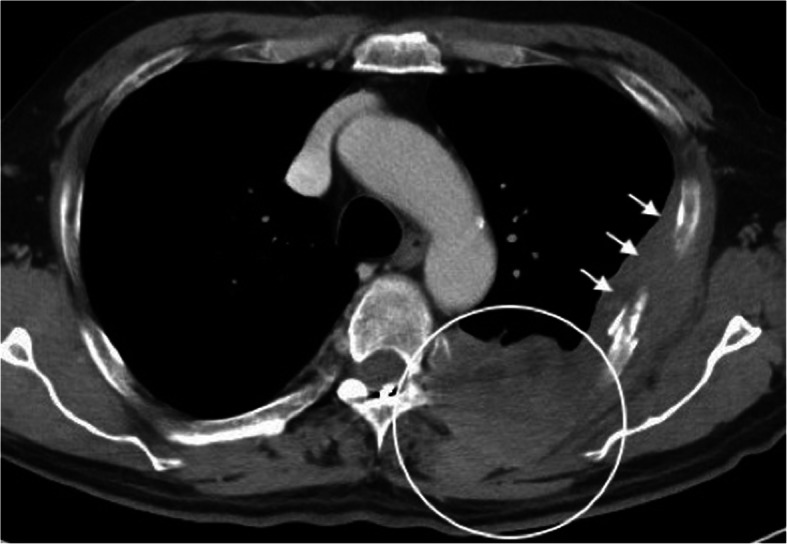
Axial slice of a CT scan showing a heterogeneous mass infiltrating the posterior thoracic tissue (in the white circle), and pleural thickening (white arrows)
